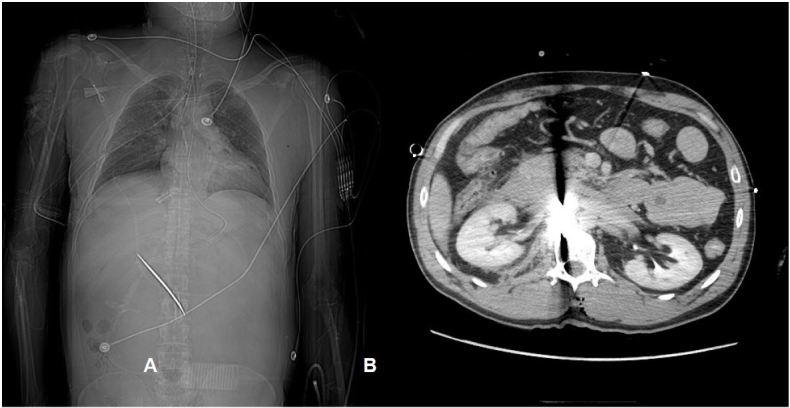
A) Scout film demonstrating retained knife blade. B) computed tomography demonstrating the retained knife blade and resulting artifact.
